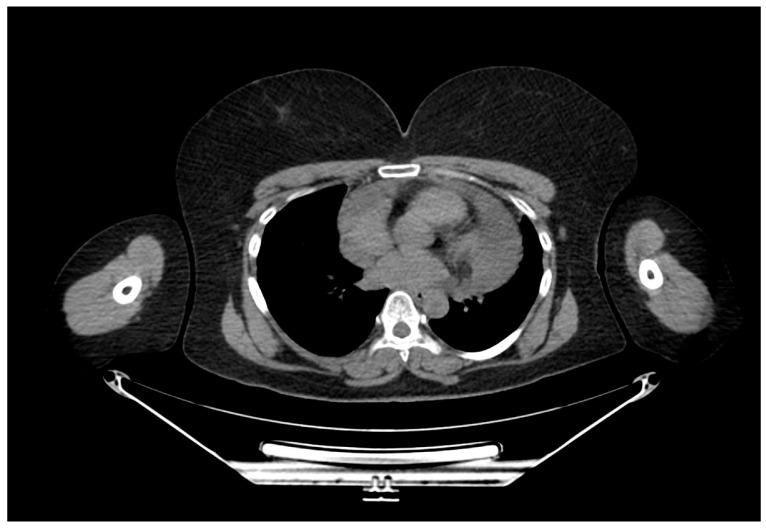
Axial view of the thoracic CT confirming massive pericardial effusion.
